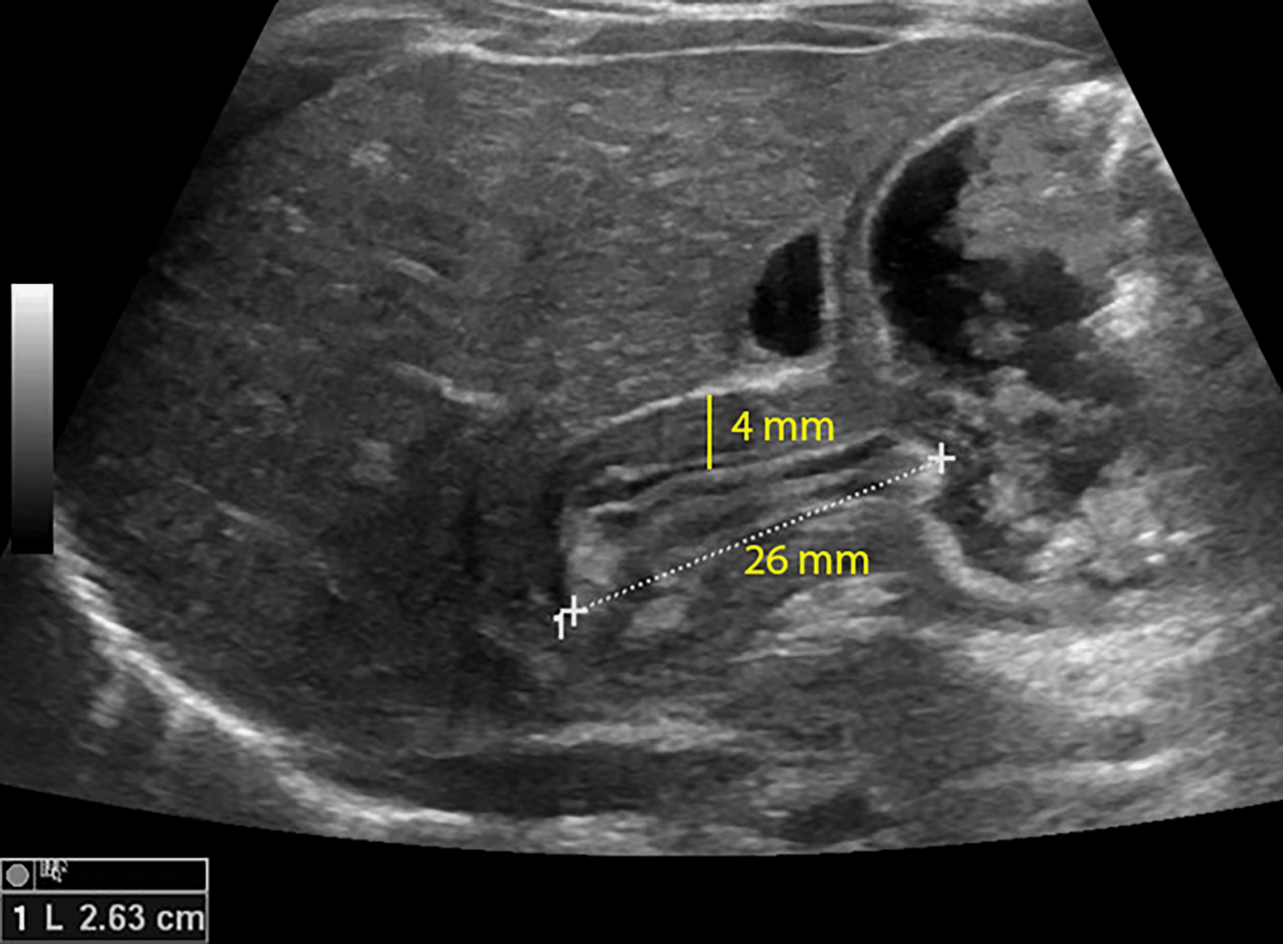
Longitudinal ultrasonography of the antropyloric region shows that an elongated and thickened pylorus spanning a length of 26 mm (white dotted line) and has a hypoechoic muscular wall measuring 4 mm in thickness (yellow line), which fulfills the criteria for the diagnosis of IHPS. IHPS, Infantile Hypertrophic Pyloric Stenosis
